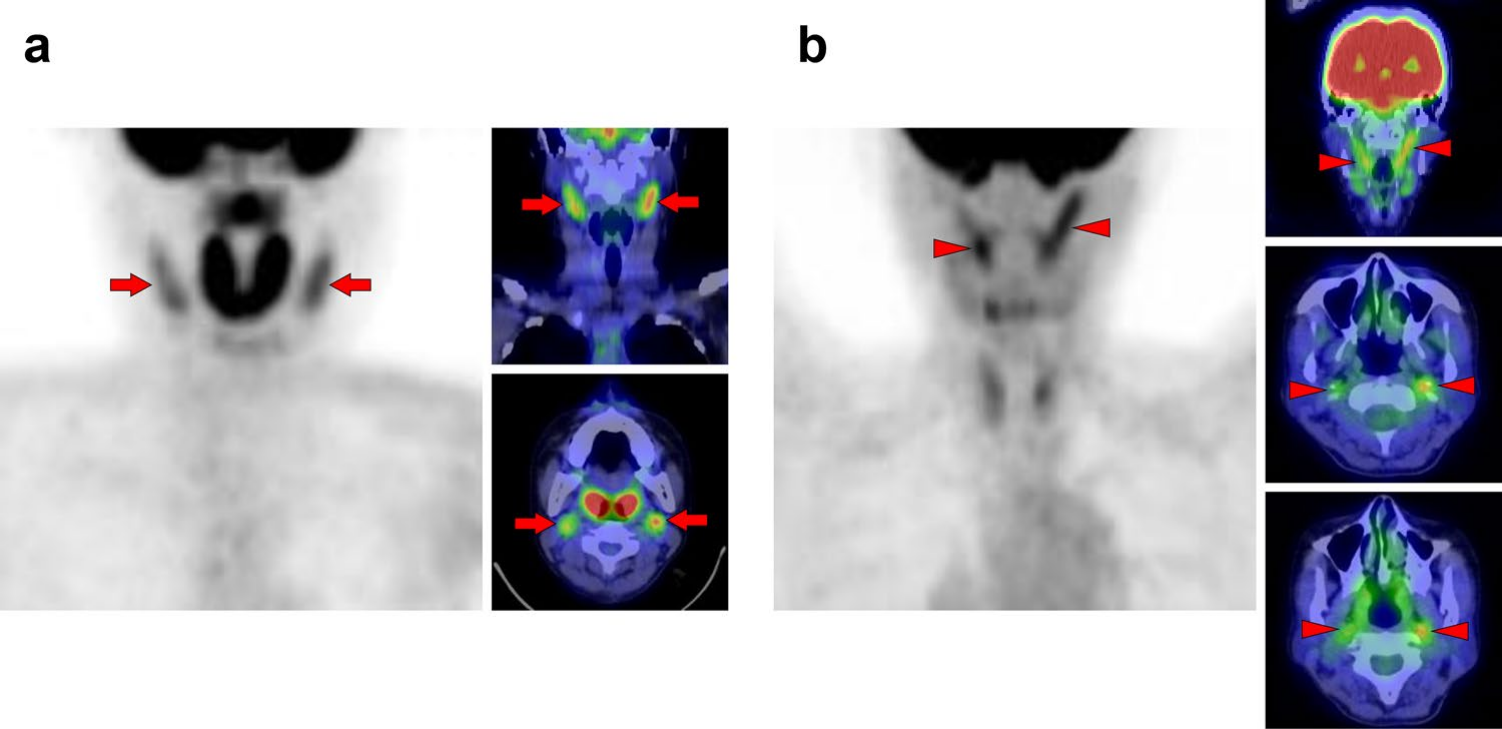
Suprahyoid muscles.* The suprahyoid muscles are located on the head side of the hyoid bone. Increased [^18^F]FDG uptake is often observed in the posterior belly of the digastric muscle (a, arrows) and the stylohyoid muscle (b, arrowheads); both belong to the suprahyoid muscle. These muscles are occasionally visualized as nodular in shape and may be misdiagnosed as a lymph-node uptake at the upper internal jugular area
